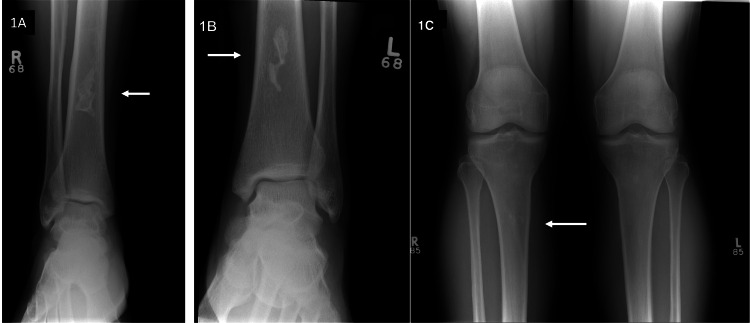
A,B) X-rays of ankles showing bilateral sclerotic lesions (white arrows) within the medullary cavity of distal tibial diaphyses, which are suggestive of intra-medullary infarcts. C) X-ray of the knees revealing a faint geographic sclerotic density within the proximal right tibial meta-diaphysis (white arrow) suspicious for small infarcts
